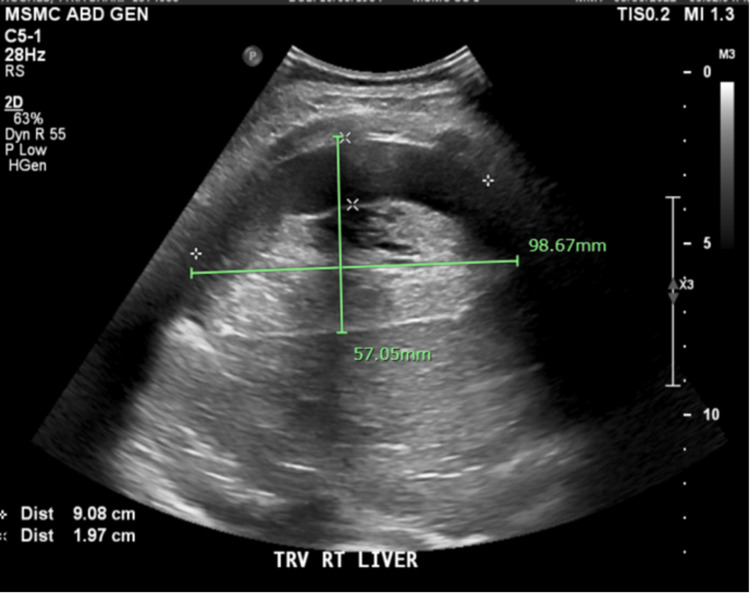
Right upper quadrant ultrasound
